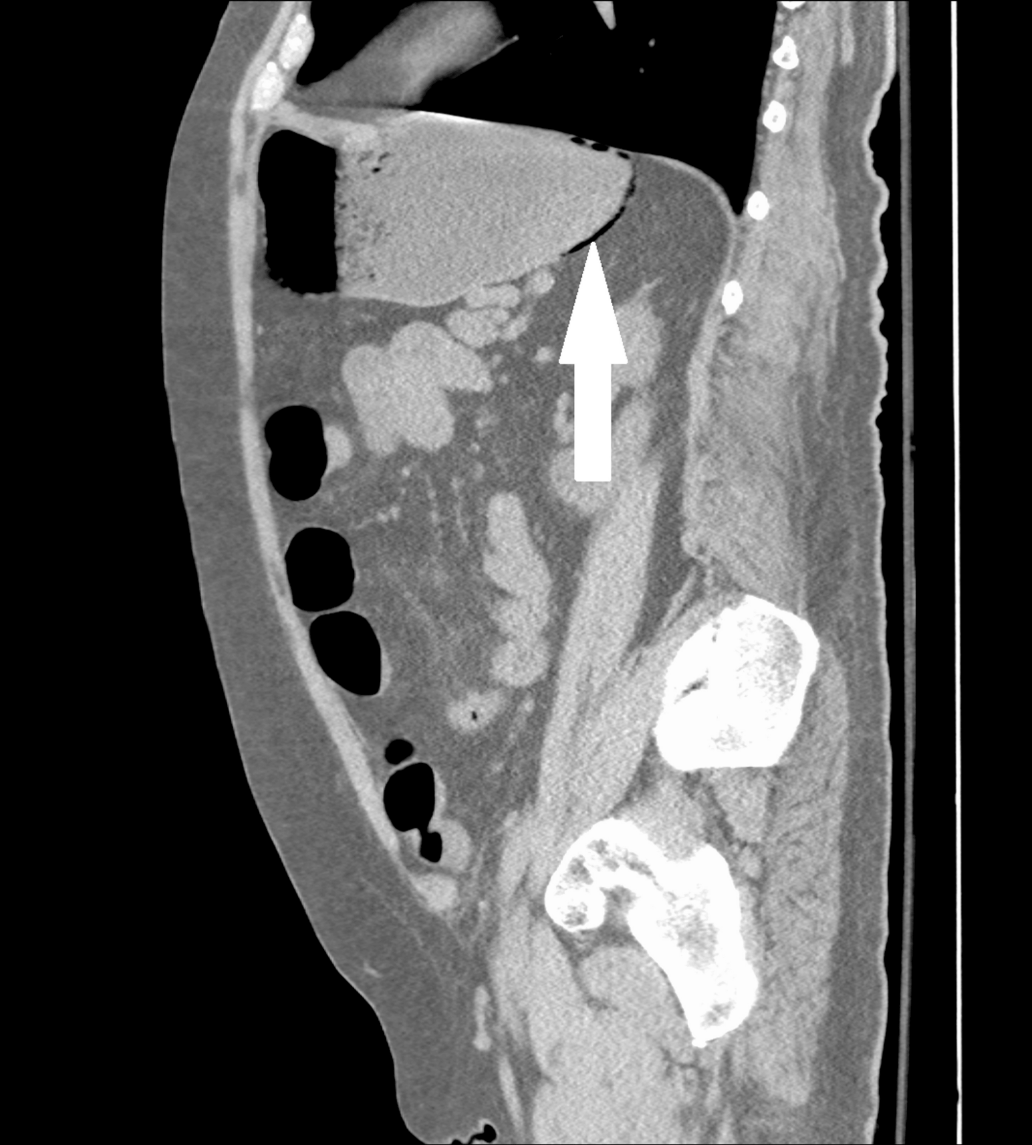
Computed tomography scan, sagittal view The normal gastric bubble of air is shown in the expected anti-dependent position, but there is an additional curvilinear tract of air (white arrows) along the posterior wall of the stomach, raising concern for gastric pneumatosis.
